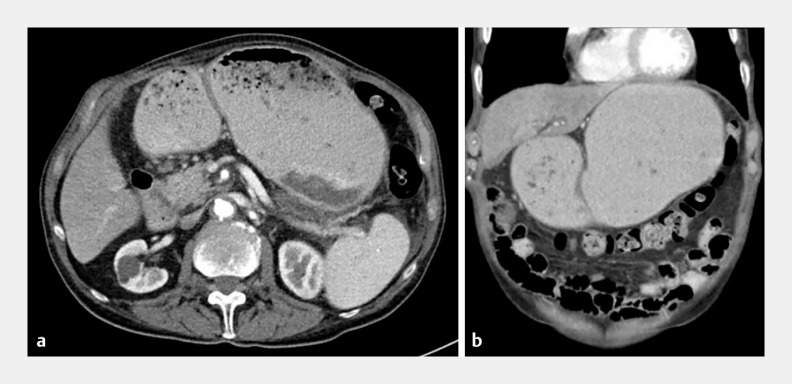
Abdominal computed tomography scan was consistent with gastric outlet obstruction.
